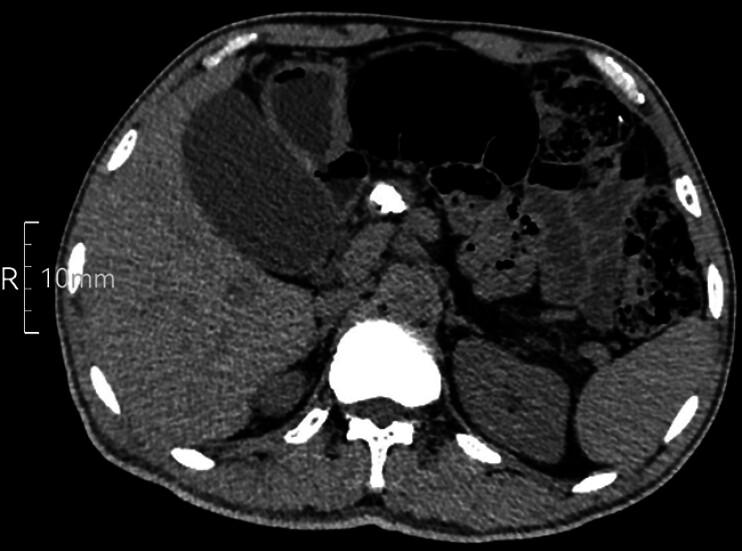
Computed tomography identified multiple radiopaque calculi within the pancreatic duct.
